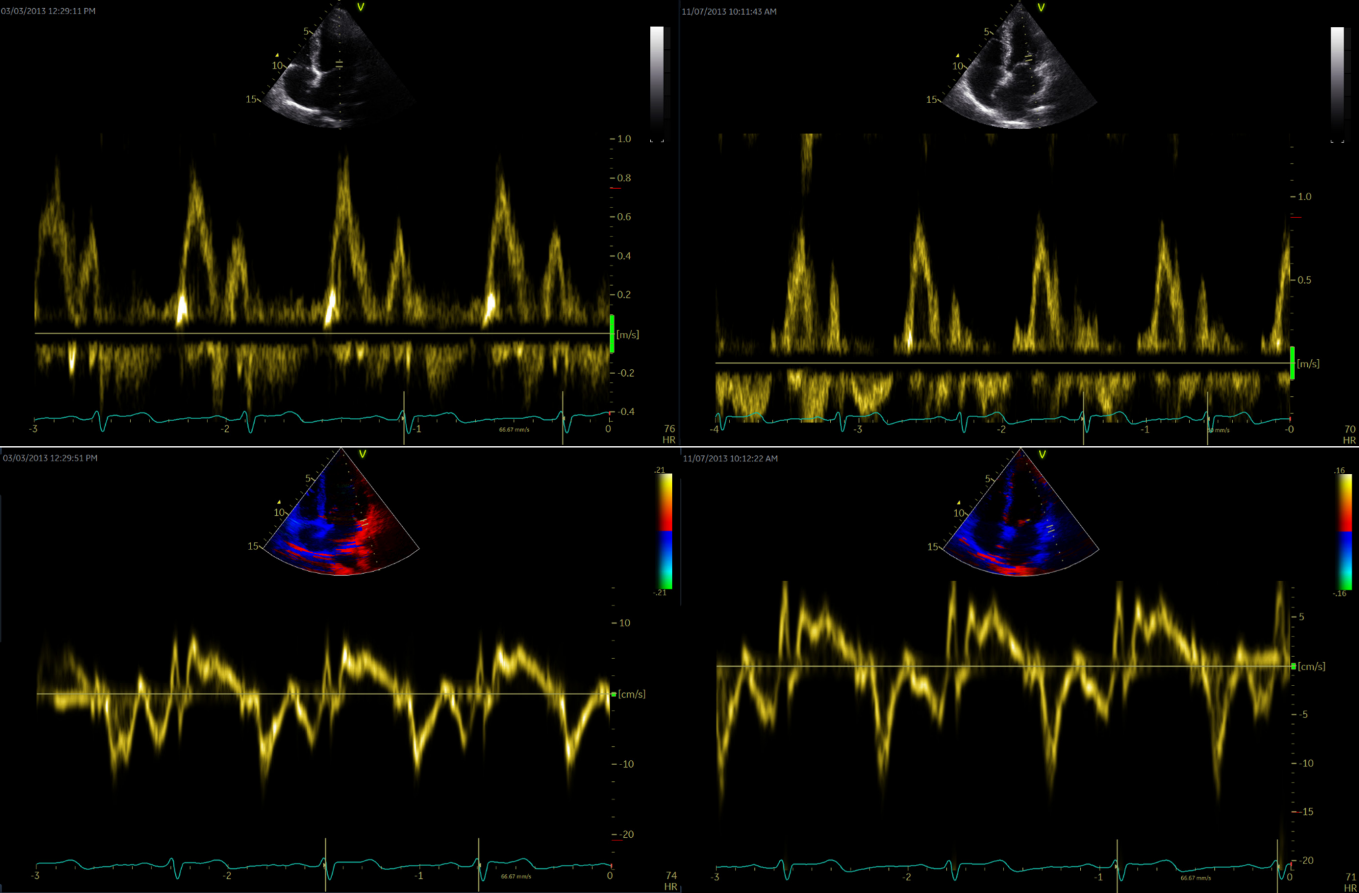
The figure shows examples of representative original Doppler echocardiographic images taken pre‐ and post‐intervention in a participant from HIS. Top: The pulsed Doppler recordings of mitral inflow pre (left) and post (right) intervention, showing early (E wave) and late (A wave) diastolic transmitral flow. Bottom: Pulsed‐wave tissue Doppler measurement at the lateral mitral annulus pre (left) and post (right) intervention, used to assess E′.
